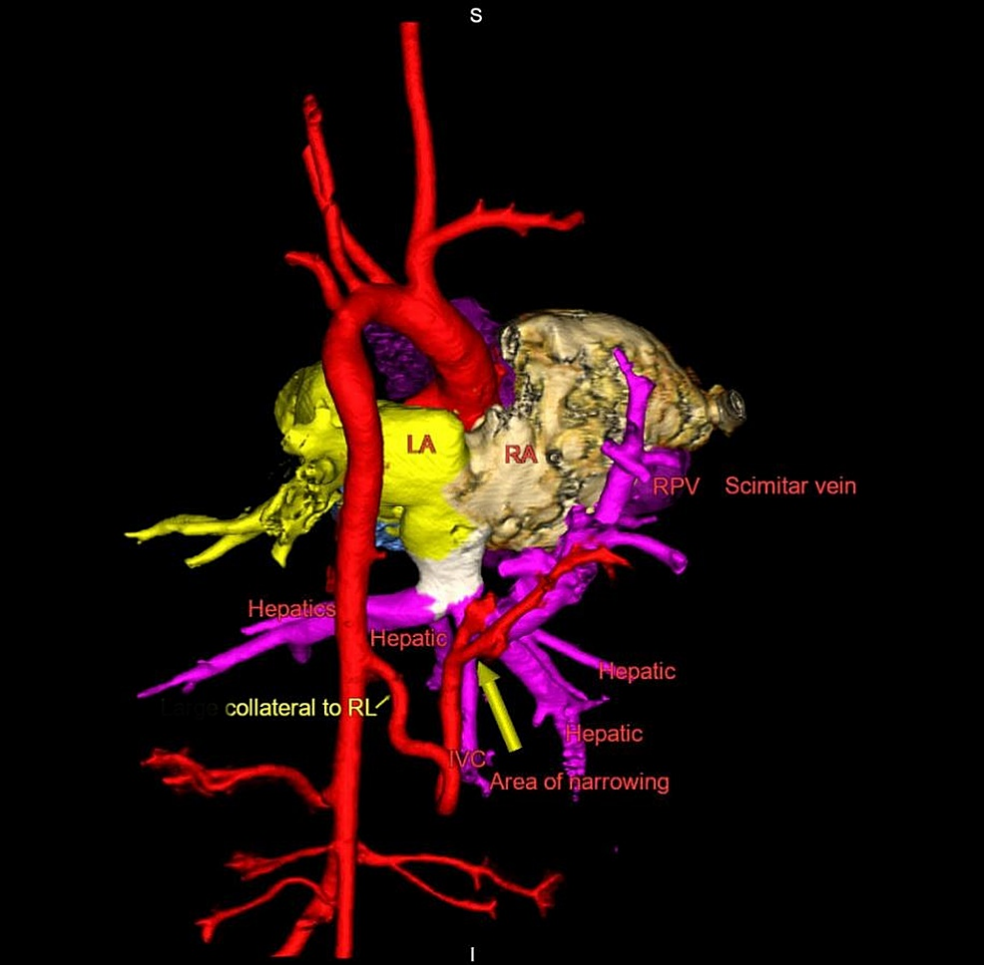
3D reconstruction of CTA images of scimitar vein, IVC, and aorta demonstrating stenosis of scimitar vein at junction with right hepatic vein and moderate-sized aortopulmonary collateral arising from descending aorta and supplying right lower lobe CTA = computed tomography angiogram, IVC = inferior vena cava, LA = left atrium, RA = right atrium, RPV = right pulmonary vein, RL = right lung.
